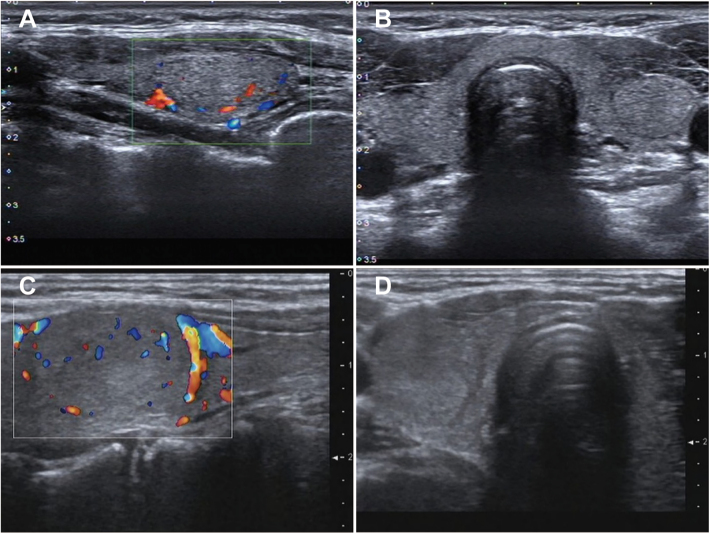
Ultrasound of a 49-year-old female patient with a left thyroid nodule. (A) Longitudinal and (B) transverse images reveal a solid nodule with a maximum diameter of 2.2 cm, regular margins, isoechogenicity and no calcifications features. Kwak-TIRADS had an indication for puncture for category 4a, while ACR-TIRADS did not for category 3 under the 2.5 cm threshold. In accordance with the serial test, FNAB for the nodule is not recommended due to the ineligibility of one of the tests. In practical terms, the patient underwent repeated punctures, both of which were Bethesda II cytopathology. A 29-year-old female patient had a nodule with a maximum diameter of 1.7 cm. (C) Longitudinal and (D) show features as follows: solid composition, isoechogenicity and regular shape without calcifications. The nodule is classified as 4b by C-TIRADS, which had indications, while it was not recommended by EU-TIRADS with category 3, which is below the puncture threshold of 2.0 cm. Likewise, the nodule could not be recommended for FNAB based on the serial test. The cytopathology was finally proven to be Bethesda II category.
